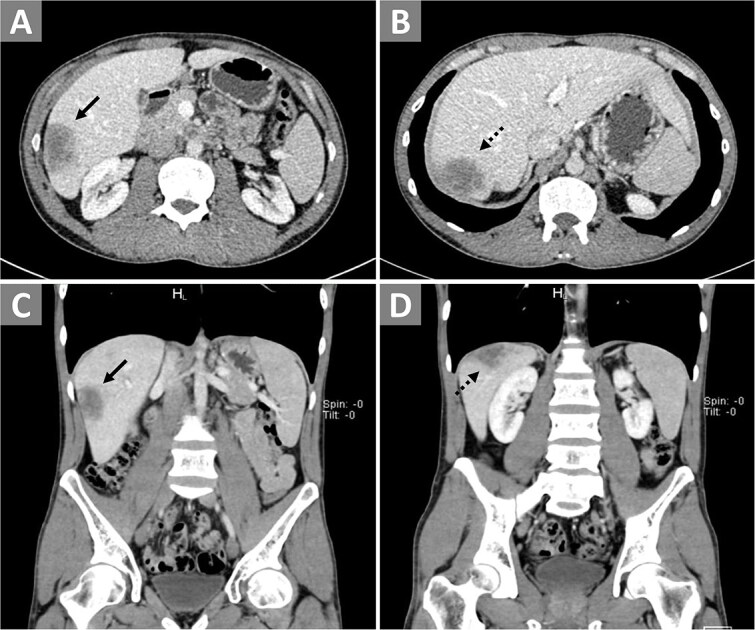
Contrast-enhanced CT of the abdomen. Panels (A) and (B) display transverse views, while panels (C) and (D) show coronal views. The solid arrows point to the lesion in the 6th segment, and dashed arrows indicate the lesion in the 7th segment of the right hepatic lobe.
